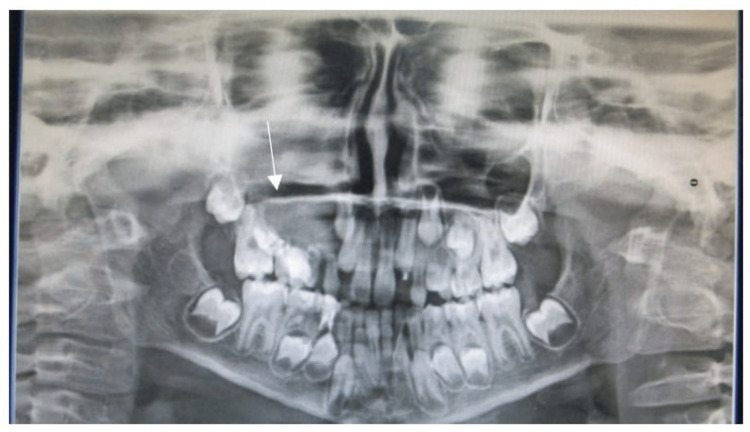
Panoramic view shows a well-defined radiolucency seen in periapical region of 54, 45, 16 region of size 2x3 cm approximately, roughly oval in shape, with well-corticated borders.
